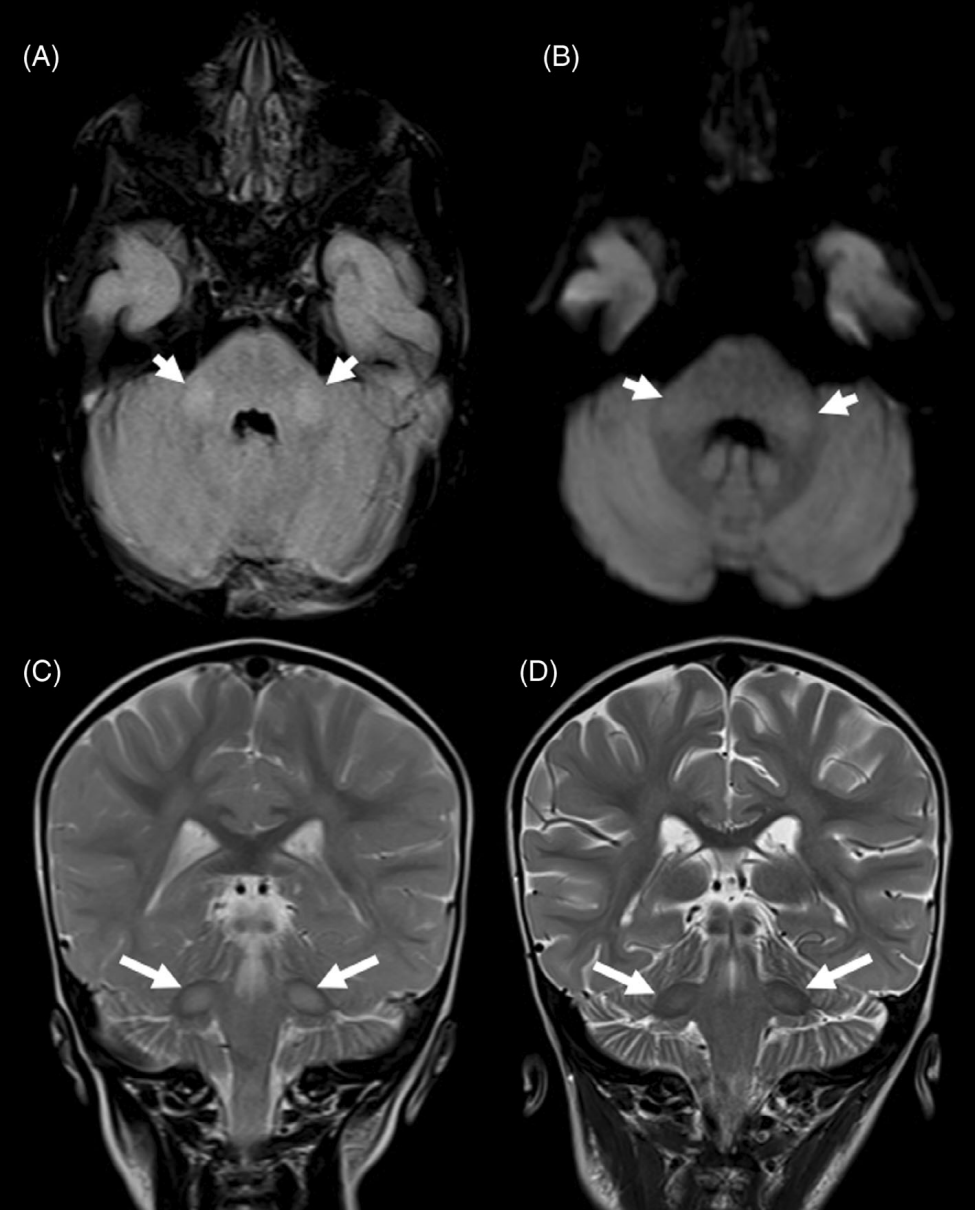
MRI of the brain before and after treatment. (A) Axial FLAIR image shows bilateral faint hyperintensities in the middle cerebellar peduncles (arrows). (B) The signal abnormalities show a faintly increased signal on diffusion‐weighted image (arrows) without diffusion restriction (the ADC map is not shown). (C) Coronal T2‐weighted image demonstrates the signal abnormality in the middle cerebellar peduncles before the treatment with vitamin B complex (arrows). (D) Interval radiological improvement of the signal abnormality in middle cerebellar pendules after treatment (arrows). Mild brain atrophy is also present.
